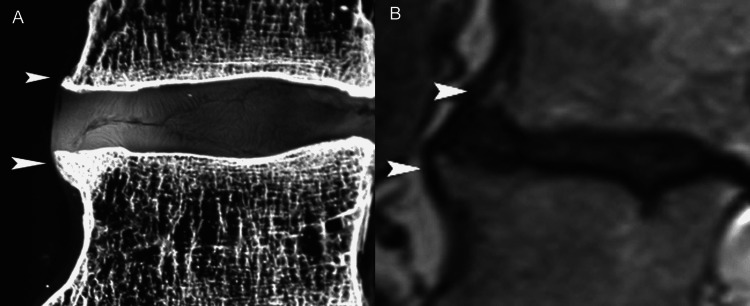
Osteophytosis. (A) Sagittal slab contact radiograph shows prominent osteophytes (arrowheads) in the margin of the vertebral bodies. (B) Sagittal MR image reveals thick cortex and fat marrow in the spurs (arrowheads).
